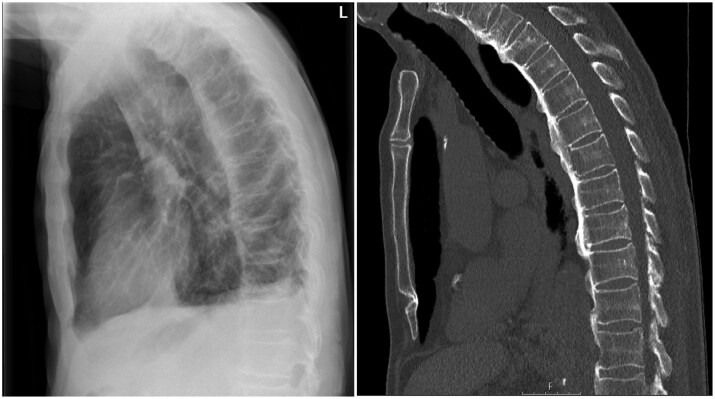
Grade III DISH on a lateral chest radiograph (left) and sagittal computed tomography scan (right) in a 55-year-old patient. Note the bridging ossification, which cannot be explained by disc degeneration. Also note the absence of facet joint ankylosis which differentiates DISH from ankylosis spondylitis
